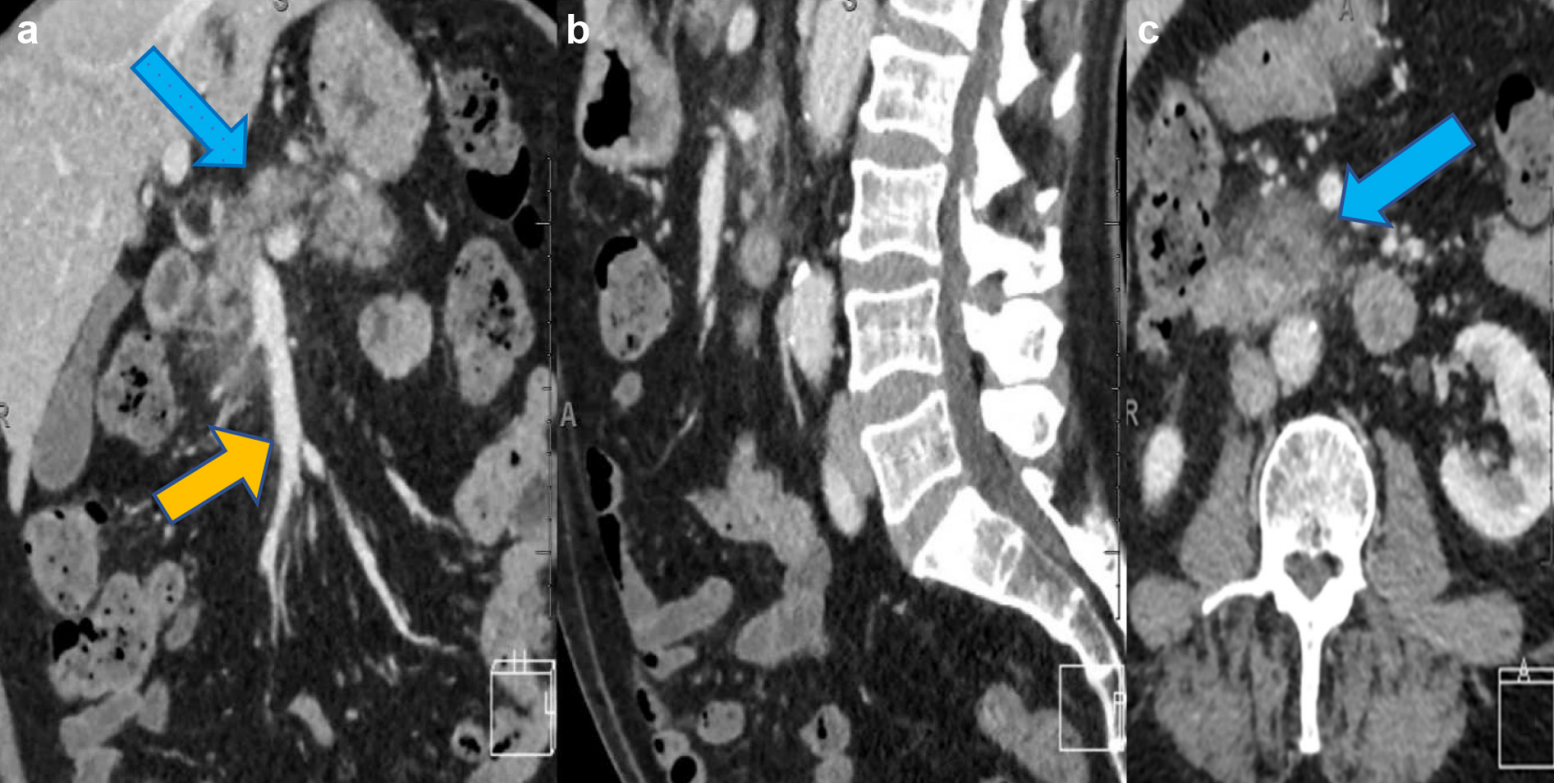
a-c, Computed tomography (CT) scans at the episode of pancreatitis 20 months before his presentation with immunoglobulin (Ig)G4-related retroperitoneal fibrosis (RPF) showing moderate peripancreatic fat stranding, particularly around the pancreatic head (*blue arrows*), with a normal-appearing aorta of normal caliber (*orange arrow*).
